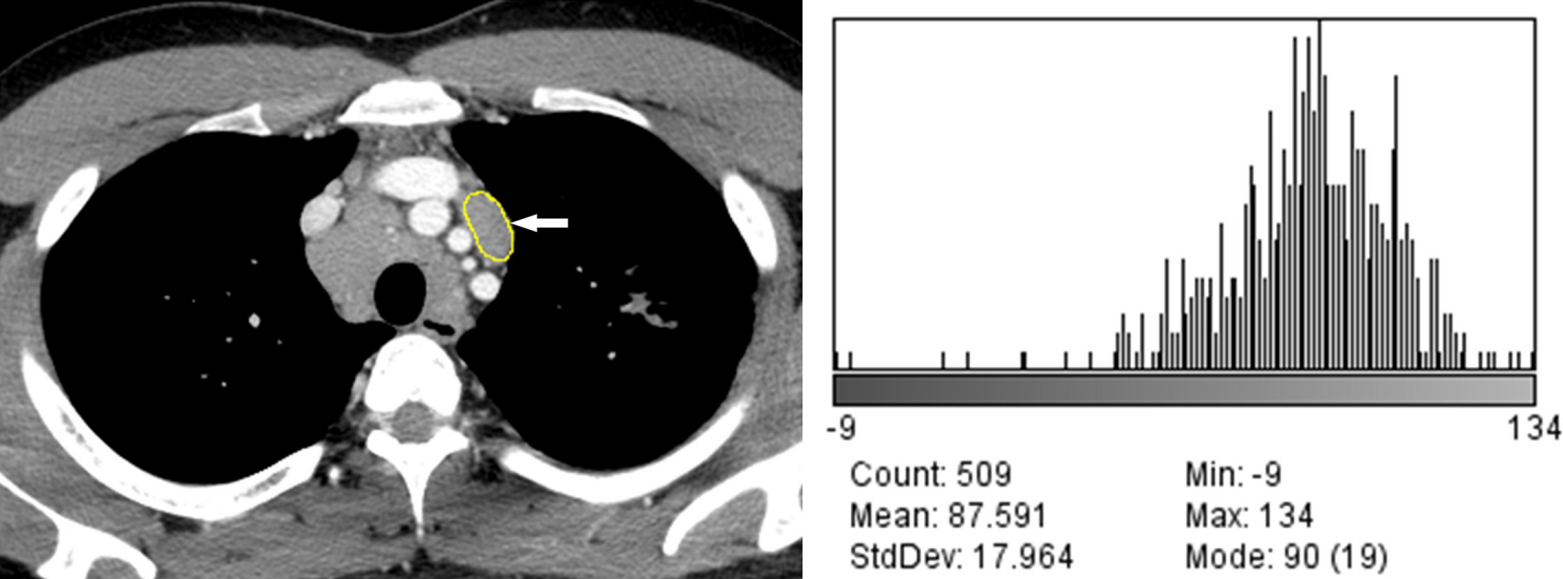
33-year-old man with sarcoidosis with lung involvement. (A) Mediastinal window image of contrast-enhanced chest CT scan shows a segmented prevascular lymph node (arrow). (B) Histogram plot shows the CT data profile of the segmented lymph node (skewness, -1.56; kurtosis, 5.26).
